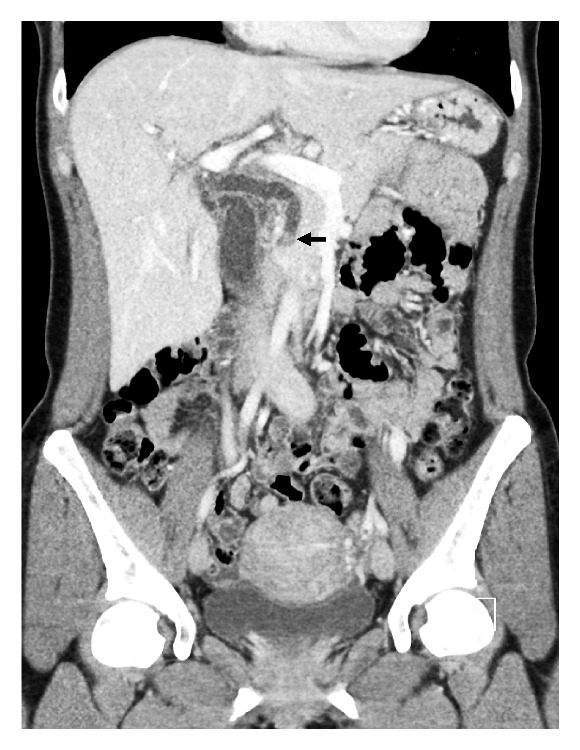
Case # 2, Dilated common bile duct with distal common bile duct stone (arrow), CT of Abdomen.
